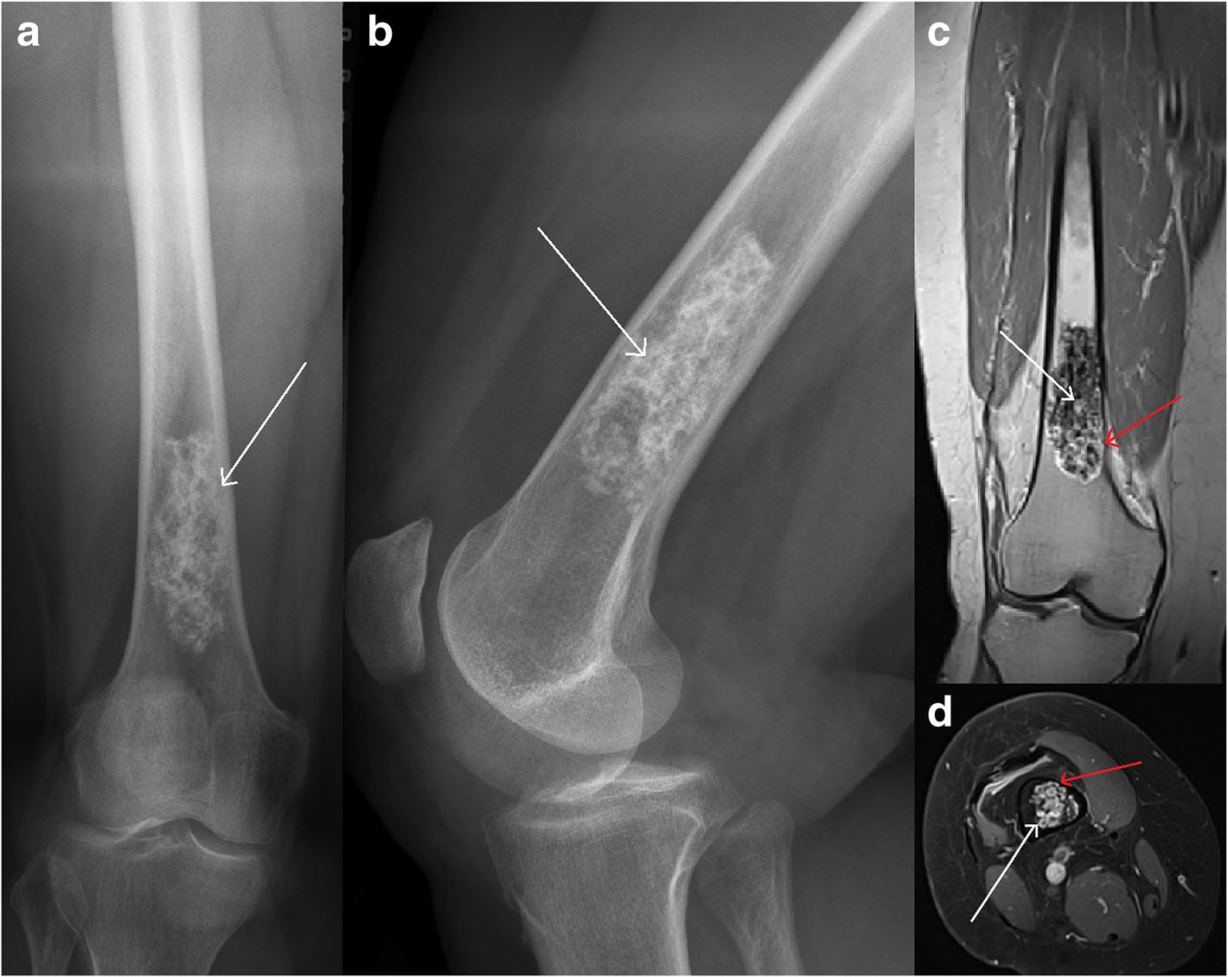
Conservative treatment at the distal femur Typical chondroid matrix (white arrows) is visible in ap (a) and lateral (b) X-rays and coronal (c) and axial (d) T1 dotarem contrast-enhanced MRI. Despite local pain, large lesion size, and endosteal scalloping (red arrows) surgery was not performed.
